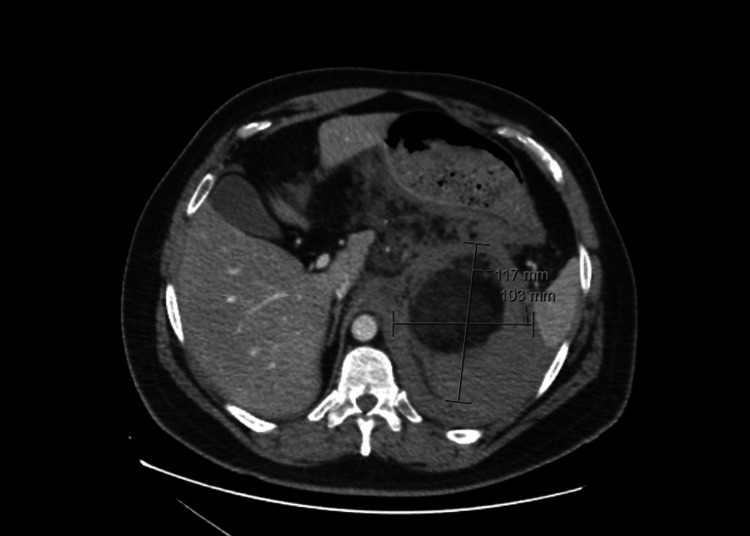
Computed tomography demonstrating renal angiomyolipoma Axial view of contrast-enhanced computed tomography of the abdomen and pelvis demonstrating a large heterogeneous left renal mass consistent with angiomyolipoma, measuring approximately 11.7 × 10.3 × 16.7 cm (calipers)
